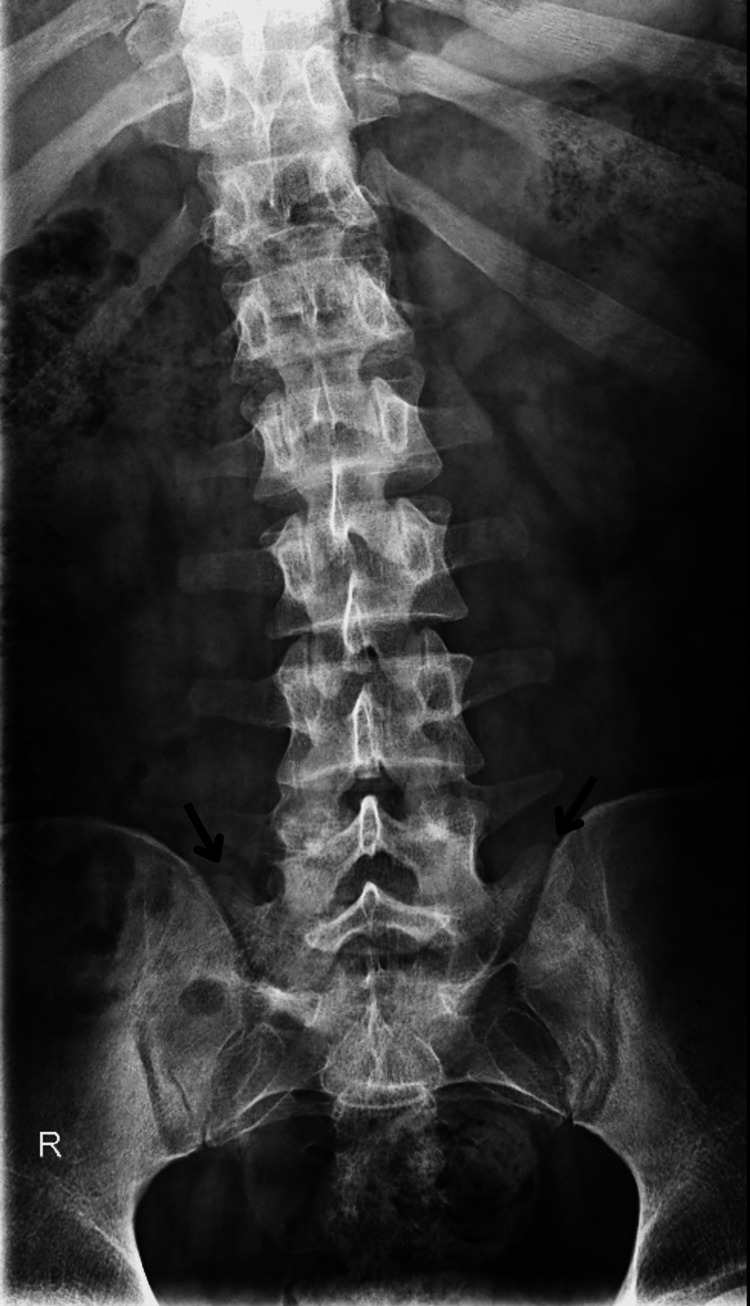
Anteroposterior lumbar spine radiograph demonstrating bilateral transitional transverse processes of the fifth lumbar vertebrae (indicated with black arrows), confirming Bertolotti's Syndrome.
